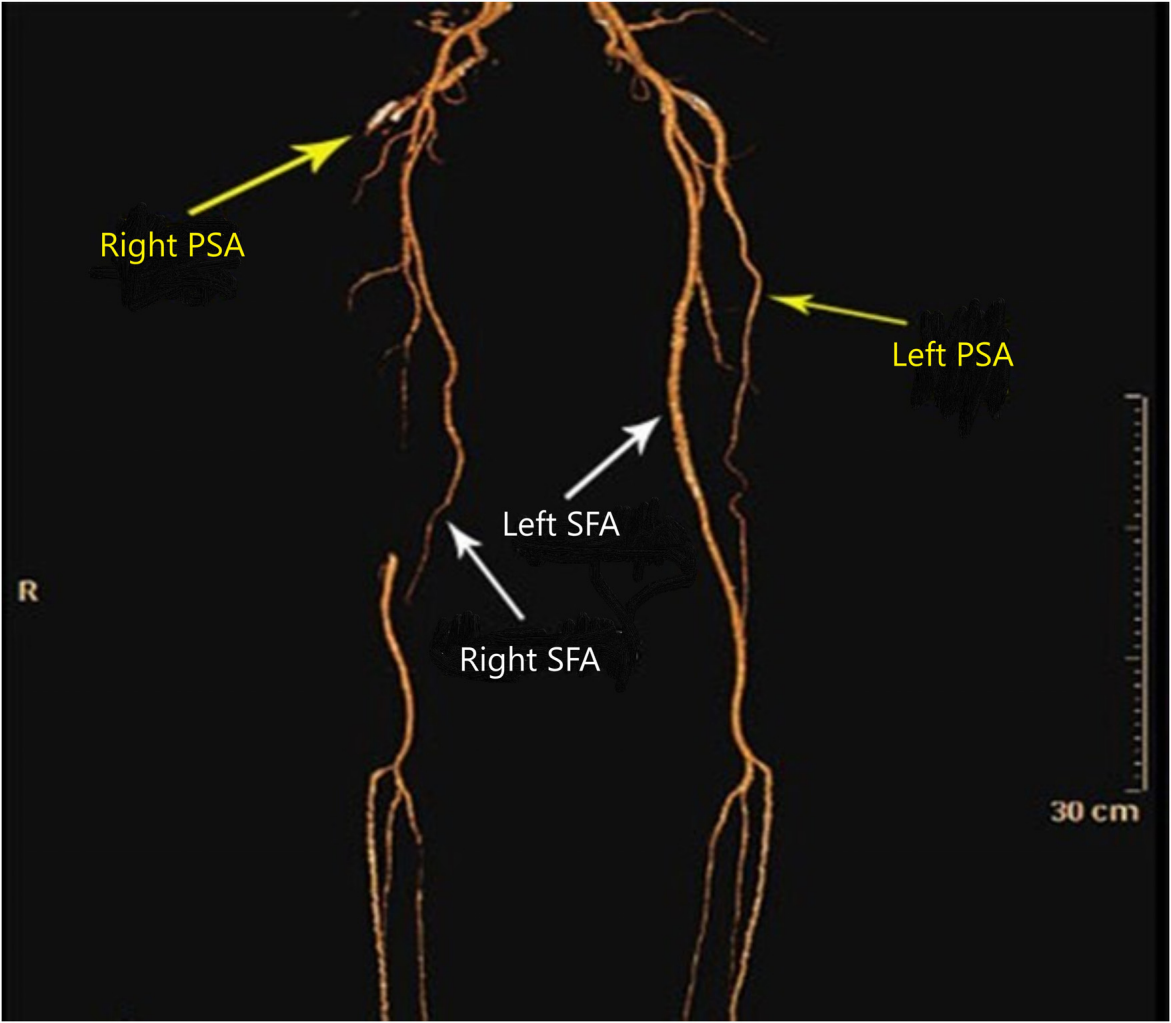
Anterior view of 3D CT reconstruction arteries. The CTA demonstrated right superficial femoral artery (SFA) was hypoplastic and no continuity to popliteal artery. The right PSA with calcified plaque and complete intraluminal thrombosis is noted. The left SFA have continuity with popliteal artery and the left persistent sciatic artery (PSA) extending downward and connected to left popliteal artery. Also, the bilateral persistent sciatic arteries are noted. (Yellow arrows).
